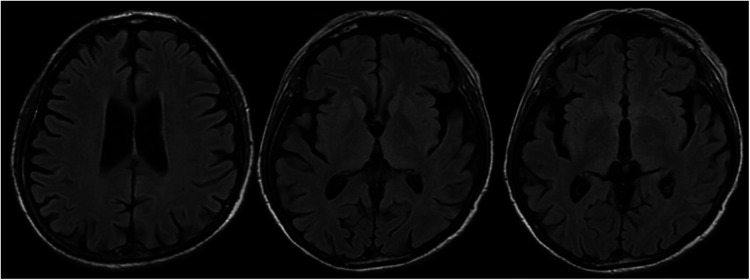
Brain FLAIR MRI. Axial section showing global cortical atrophy, mainly in the right temporal lobe.
